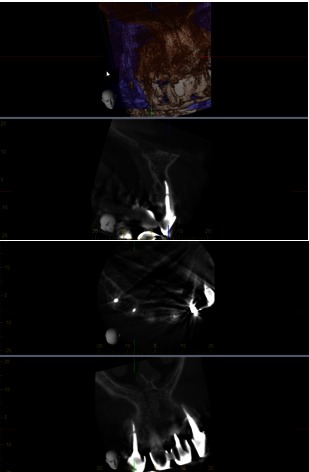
Fig. 6CBCT 5 years follow up
